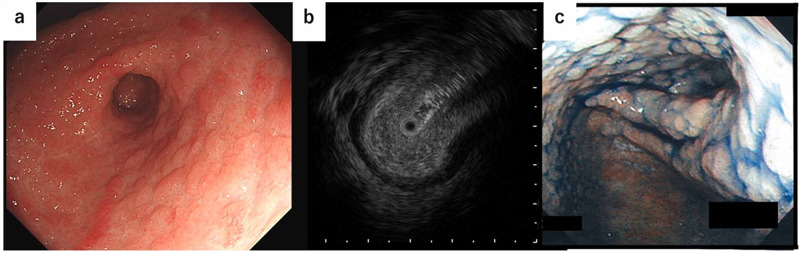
Endoscopic image in the gastric antral portion a. Endoscopic image demonstrating mucosal edema and thickening accompanied with scattered reddish patchy flat elevated lesions in the gastric antral portion. b. Endoscopic ultrasonography showing marked thickening of the antral submucosal layer, though no thickening of the muscular layer or accumulation of peripheral effusion was observed. c. Endoscopic image of the antral portion following indigo carmine spraying obtained at a nearby clinic two years prior. The results indicated a circumferential narrowing of the antral portion, similar to the finding in our examination
